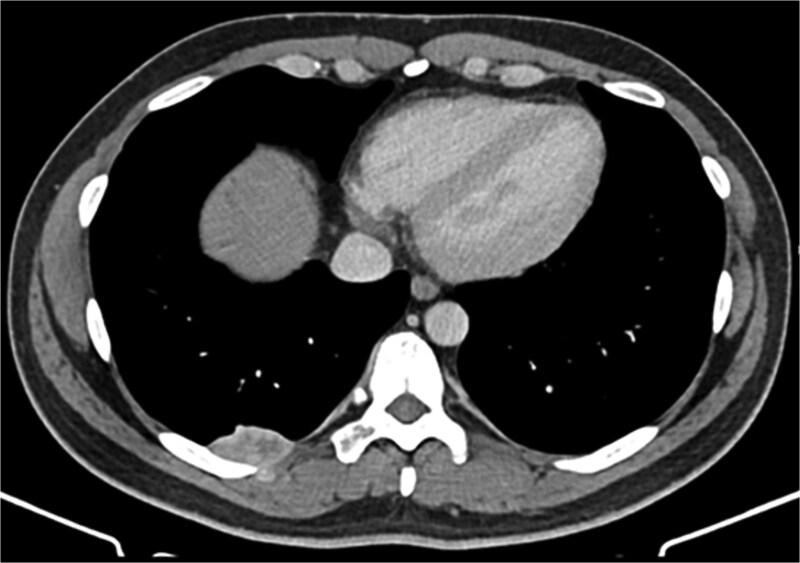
Contrast-enhanced CT scan of the chest*.*
